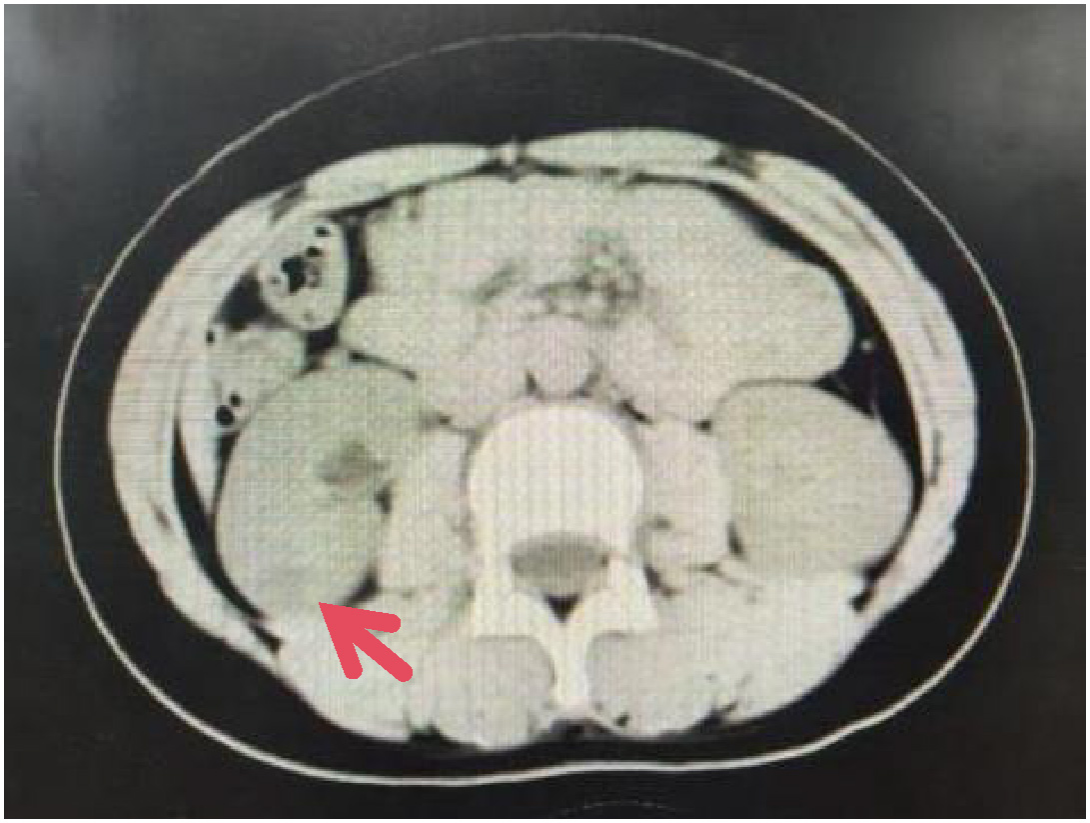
Kidney CT scan with contrast enhancement.
